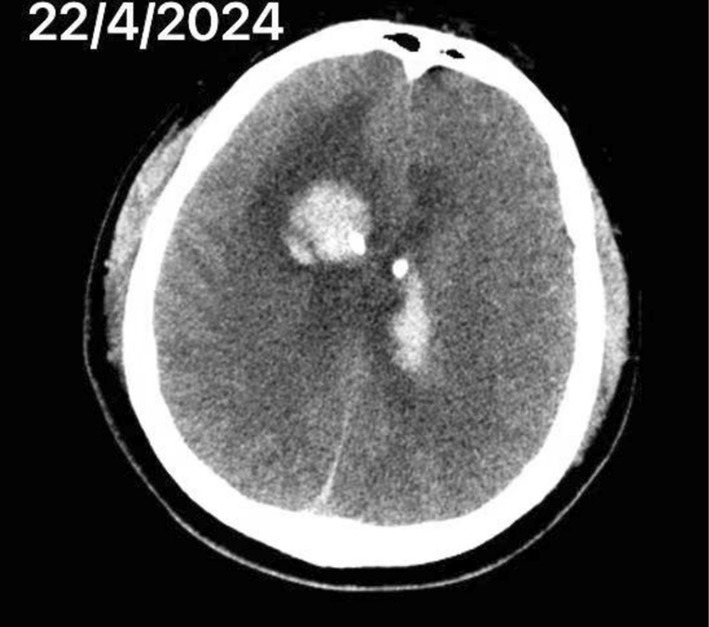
CT of the head on April 22, 2024.
